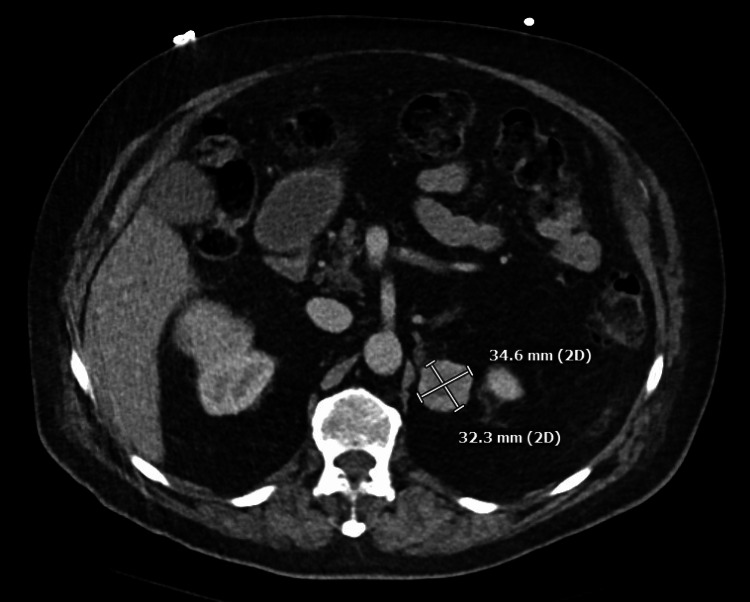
CT abdomen pelvis demonstrated a 3.5 x 3.2 x 3.4 cm soft tissue lesion adjacent to the superior pole of the left kidney and posterior to the left adrenal gland. CT: computed tomography
